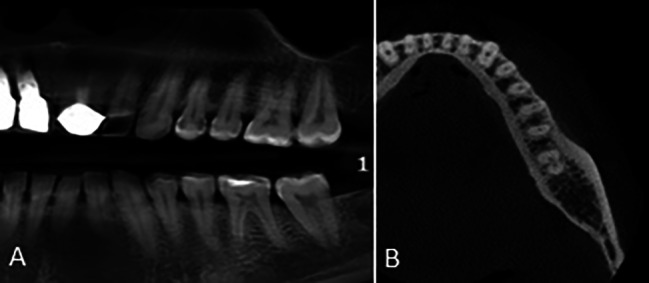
Mandibular second molar tooth with (A) type 3 morphology on the panoramic-like image and (B) C-shaped pattern (type C3) on the axial CBCT section
